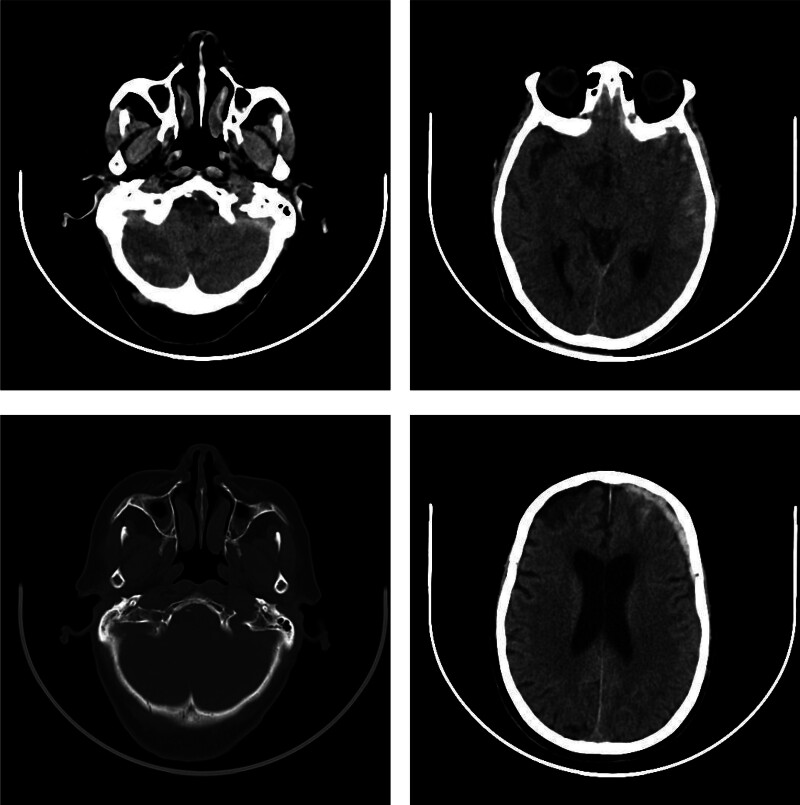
Head CT image of the patient admitted to the emergency department. CT = computed tomography.
